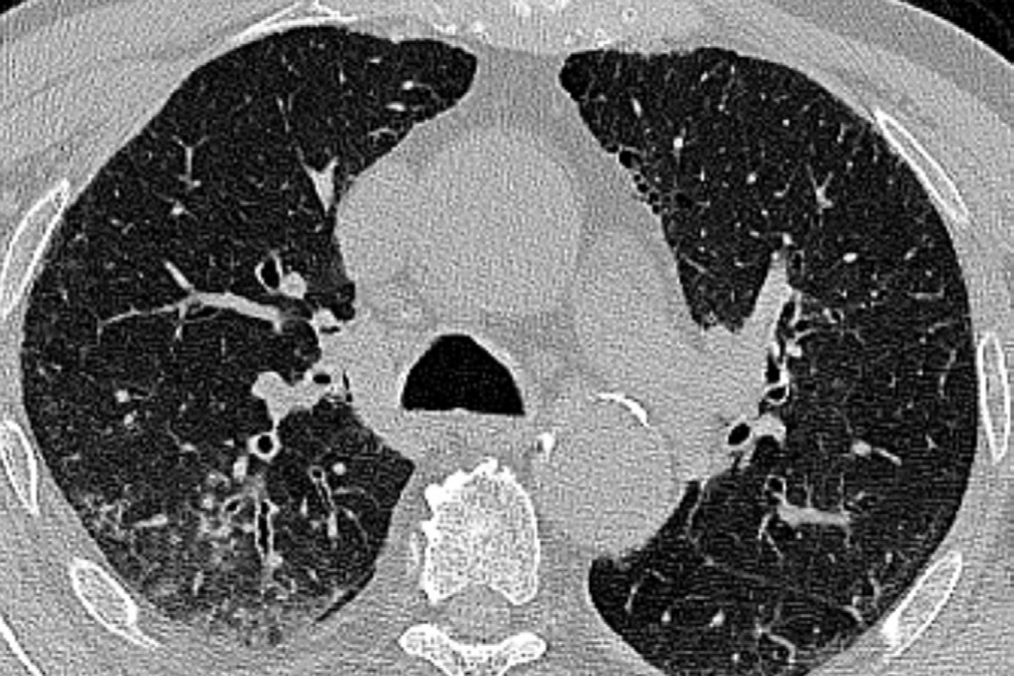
Axial CT image of a 78-year-old male Covid-19 patient (α variant), showed tree-in-bud opacities in right upper lobe with bronchial wall thickening.
